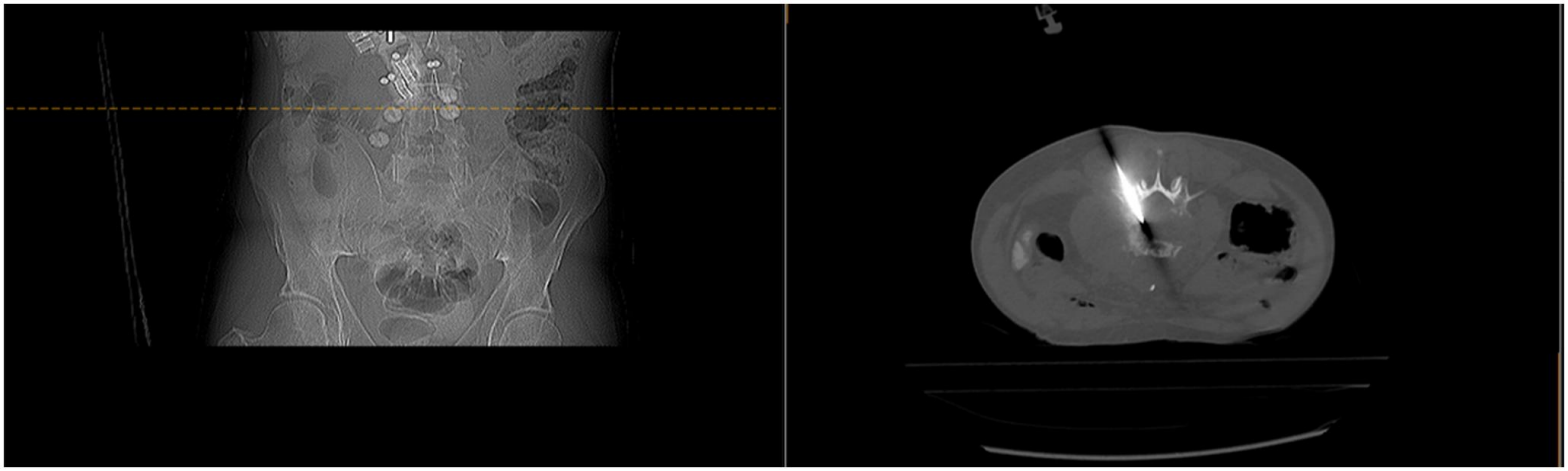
Robotic assisted biopsy of an L4 lesion under CT guidance using a table-mounted robotic system (micromate/interventional systems).
